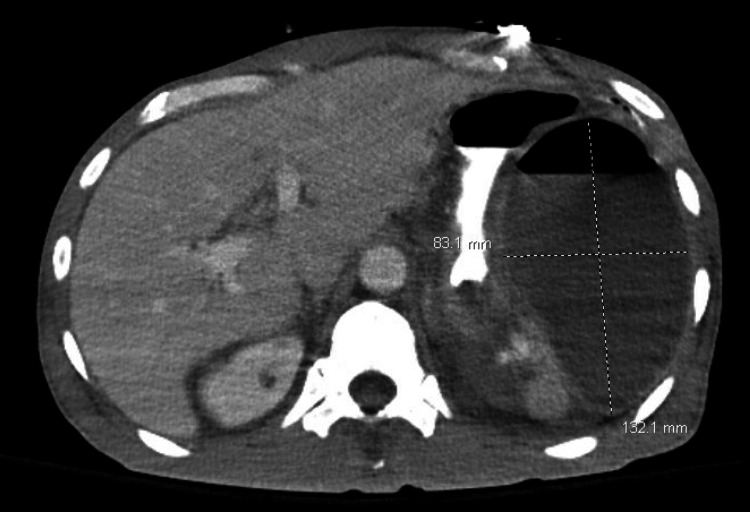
A computed tomography scan of the patient's abdomen in a sagittal view. The perisplenic abscess is marked using white lines.
